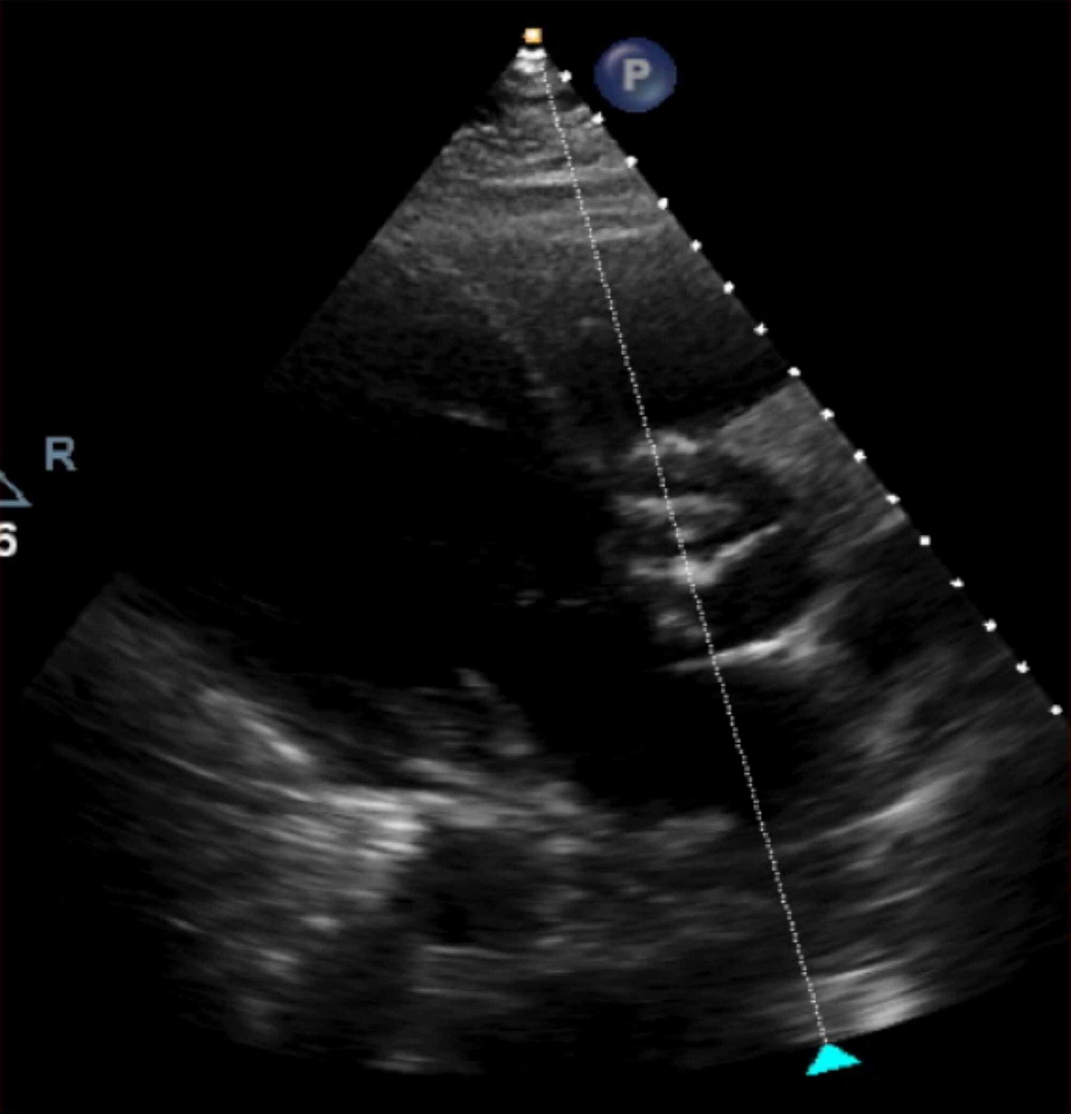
Transthoracic echocardiogram showing bicuspid aortic valve (BAV)
